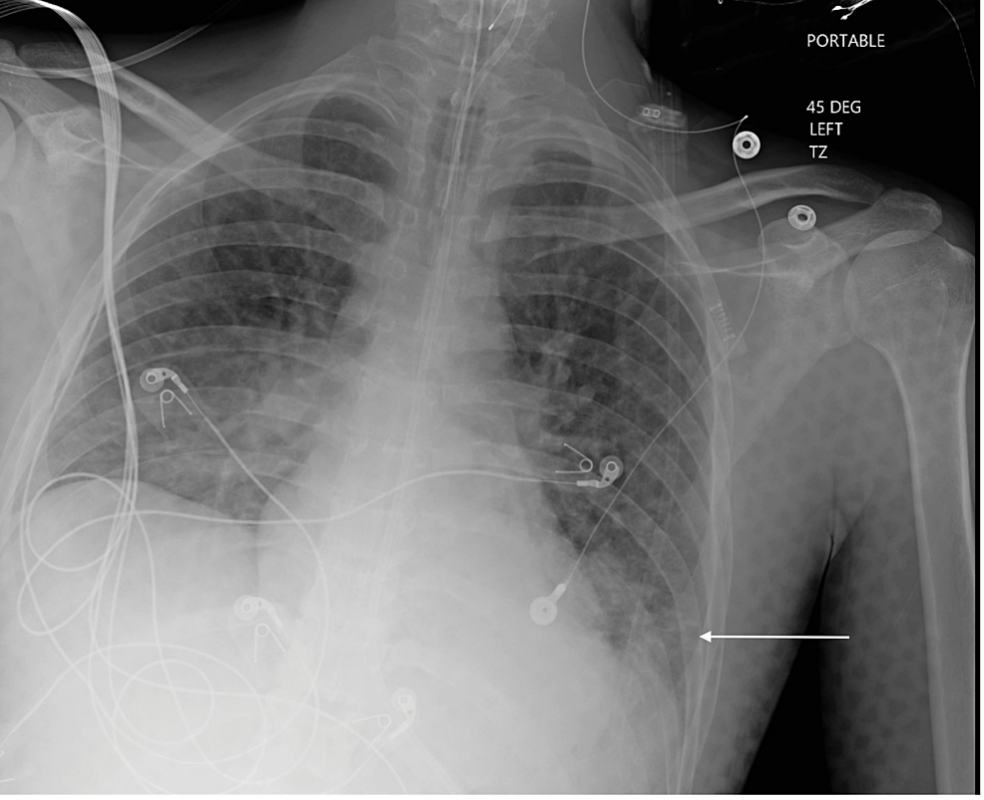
Chest X-ray revealed confluent airspace opacity in the left lower lobe likely representing pneumonia or atelectasis.
